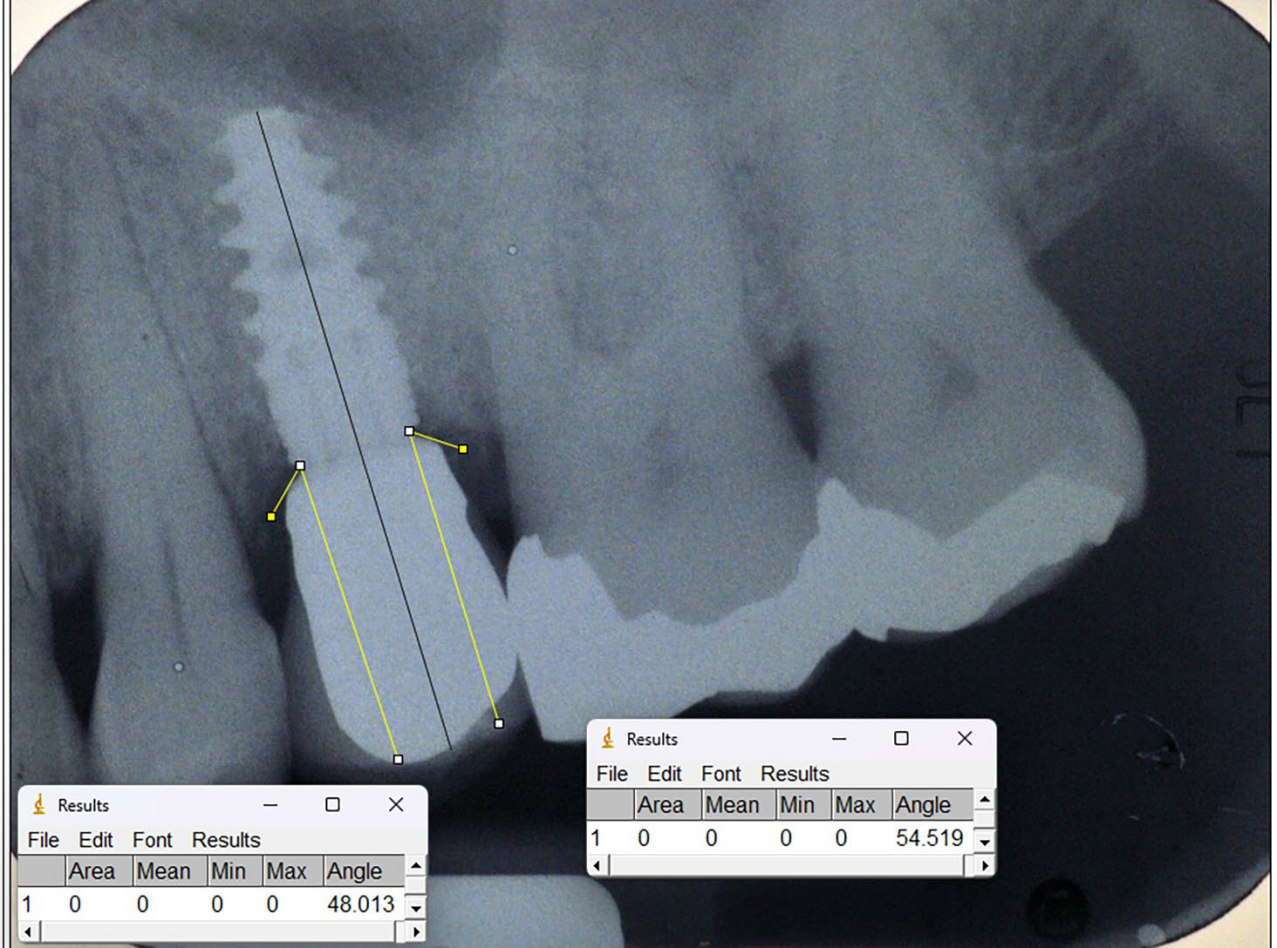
Example of prosthetic emergence angle assessed on the radiograph (mesial and distal).
